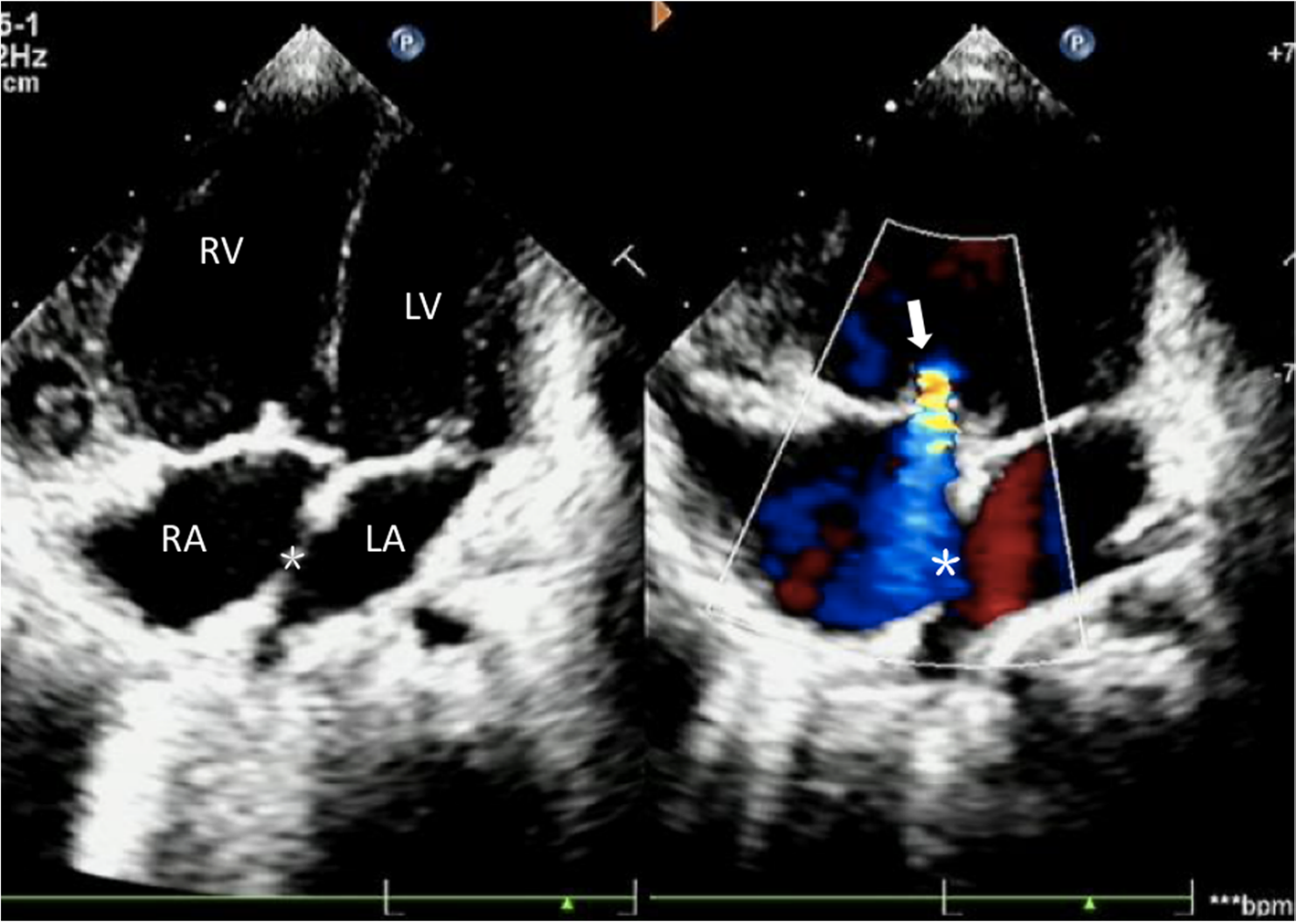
Transthoracic echocardiogram. The four chamber view of cardiac ultrasound shows that the right heart was significantly enlarged,“*”indicates atrial septal defect, the shunt signal of blood flow from right to left can be seen at the defect, "↓" indicates moderate to severe tricuspid regurgitation. LV=left ventricular; LA=left atrial; RV=right ventricular; RA=right atrial
